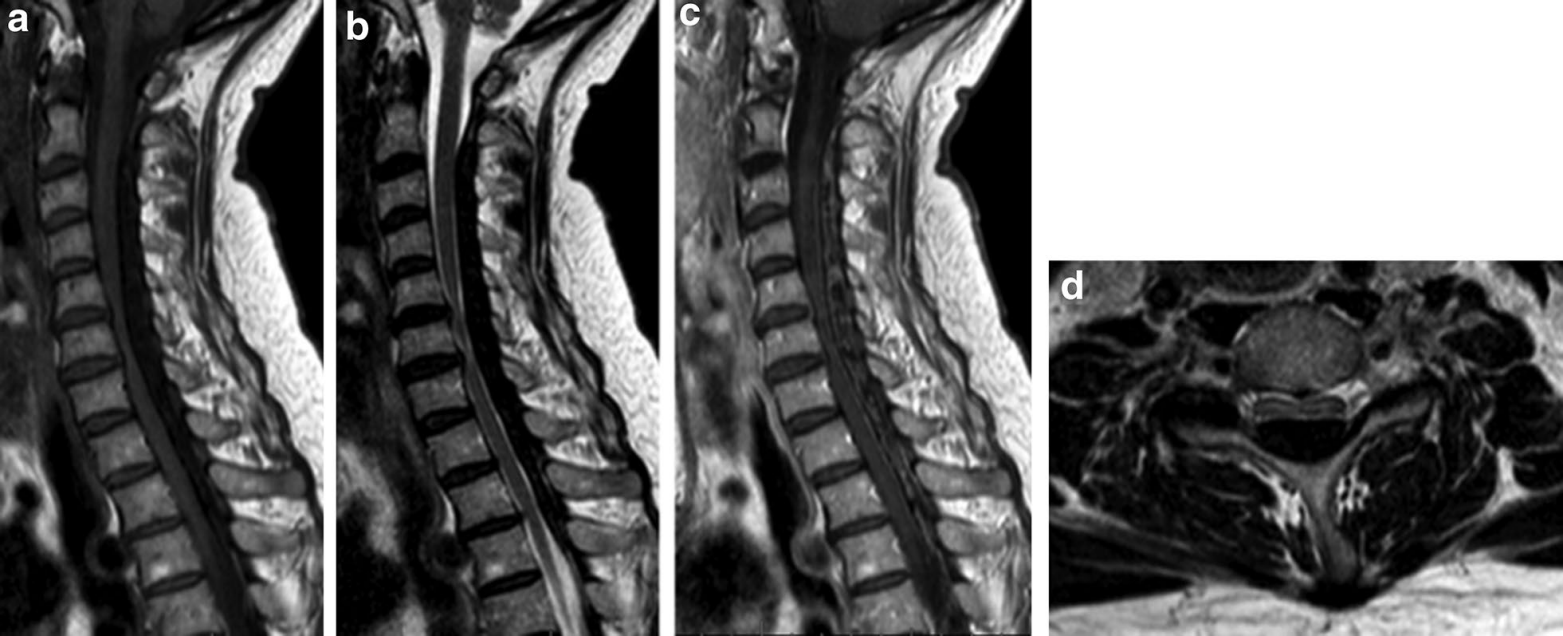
Sagittal MRI in T1- (a) and T2- (b) weighted sequences. c T1-weighted sequence with heterogeneous gadolinium uptake. d Extensive spinal cord compression at C6
